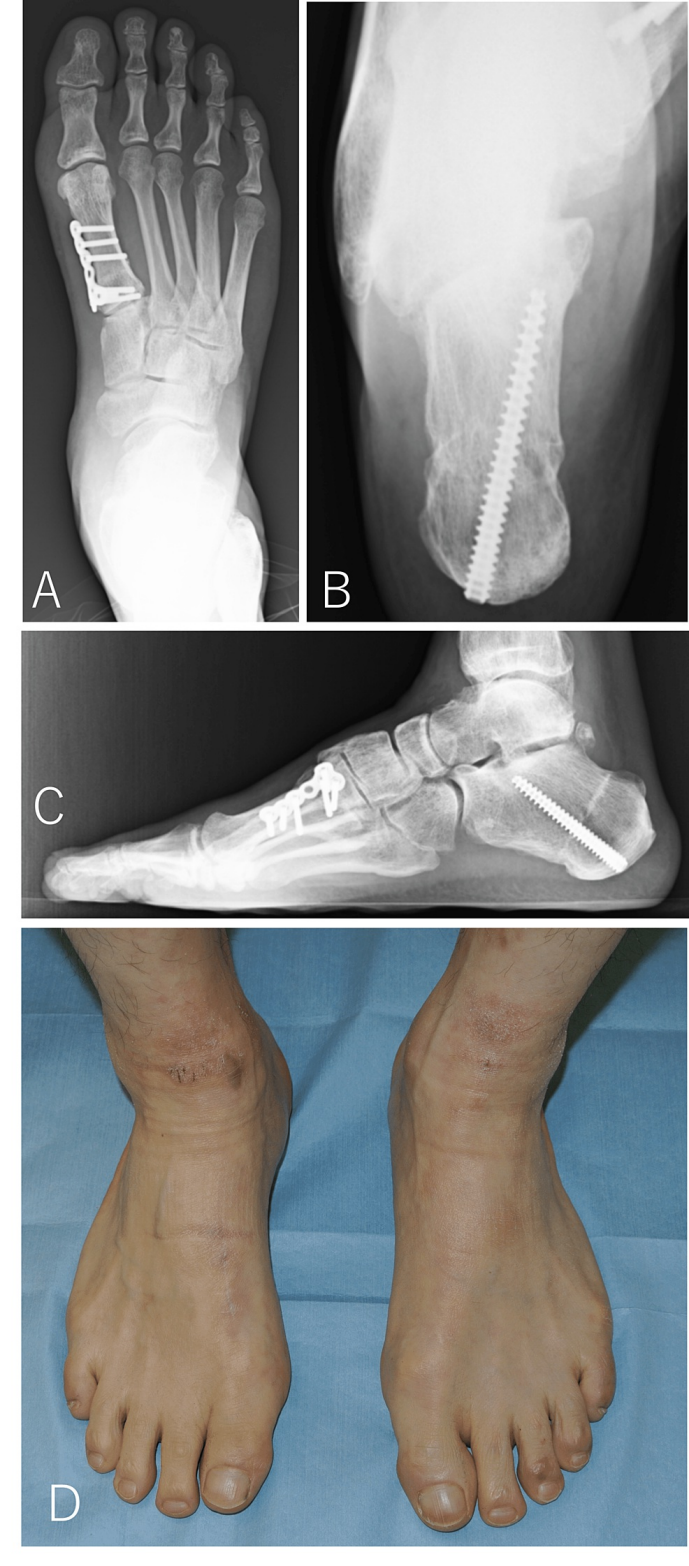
Plain radiographs and photograph one year after surgery (A) Dorsoplantar view. (B) Lateral view. (C) Axial view of the calcaneus. (D) Clinical photo.
